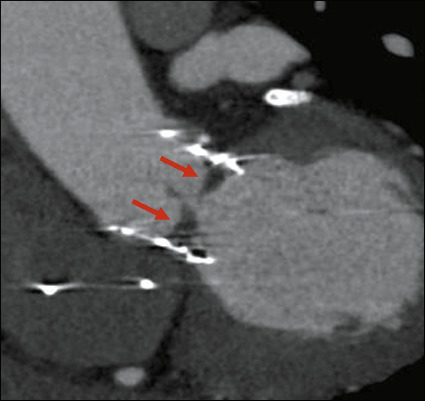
CTA of an 81-year-old male patient who had undergone TAVR one month earlier, showing
